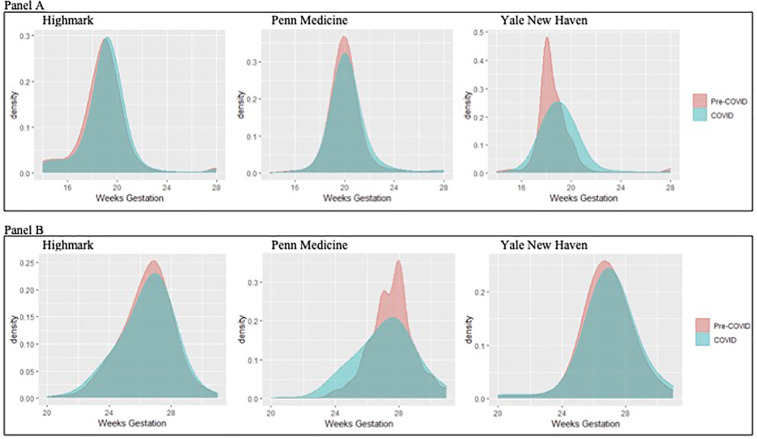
Distribution of completion of second trimester testing with an ultrasound to assess fetal anatomy (panel A) and gestational diabetes (panel B) across the three data sources.
